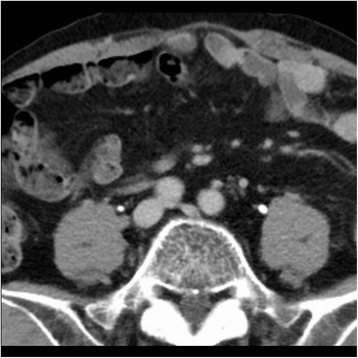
Contrast-enhanced computed tomography at 3 months after the initiation of anticoagulation therapy. The thrombosis was disappeared completely
